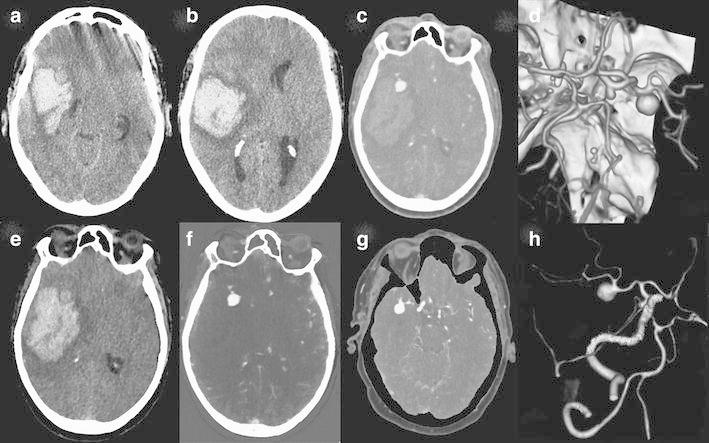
60-year-old male presented with right temporal lobe intracranial hemorrhage. Initial NECT (*a*, *b*) showed large ICH with mass effect. Mixed images (*c*) of DECTA revealed a right middle cerebral artery (MCA) aneurysm. VNC (*e*) shows the hematoma and the denser aspect of the aneurysm as also is present on the NECT. At the IOM (*f*) the aneurysm is appreciated, but not the hematoma. (*g*) shows bone-removed (BR) DECTA. VR CTA of Willis before BR (*d*) and after BR (*h*) depicts MCA aneurysm and related vessels. Occlusion of the left ICA is better visible on BR-VR, a second aneurysm is present at the posterior communicating artery. All images are deprived from one dataset. DECTA 80/Sn140 kVp, 310/155 mAs, CTDI 26.37 mGy, DLP 454 mGycm; 95 cc iodinated contrast 300 mg/ml; injection rate 5.5 cc/s; 40 cc saline flush 5.5 cc/s
